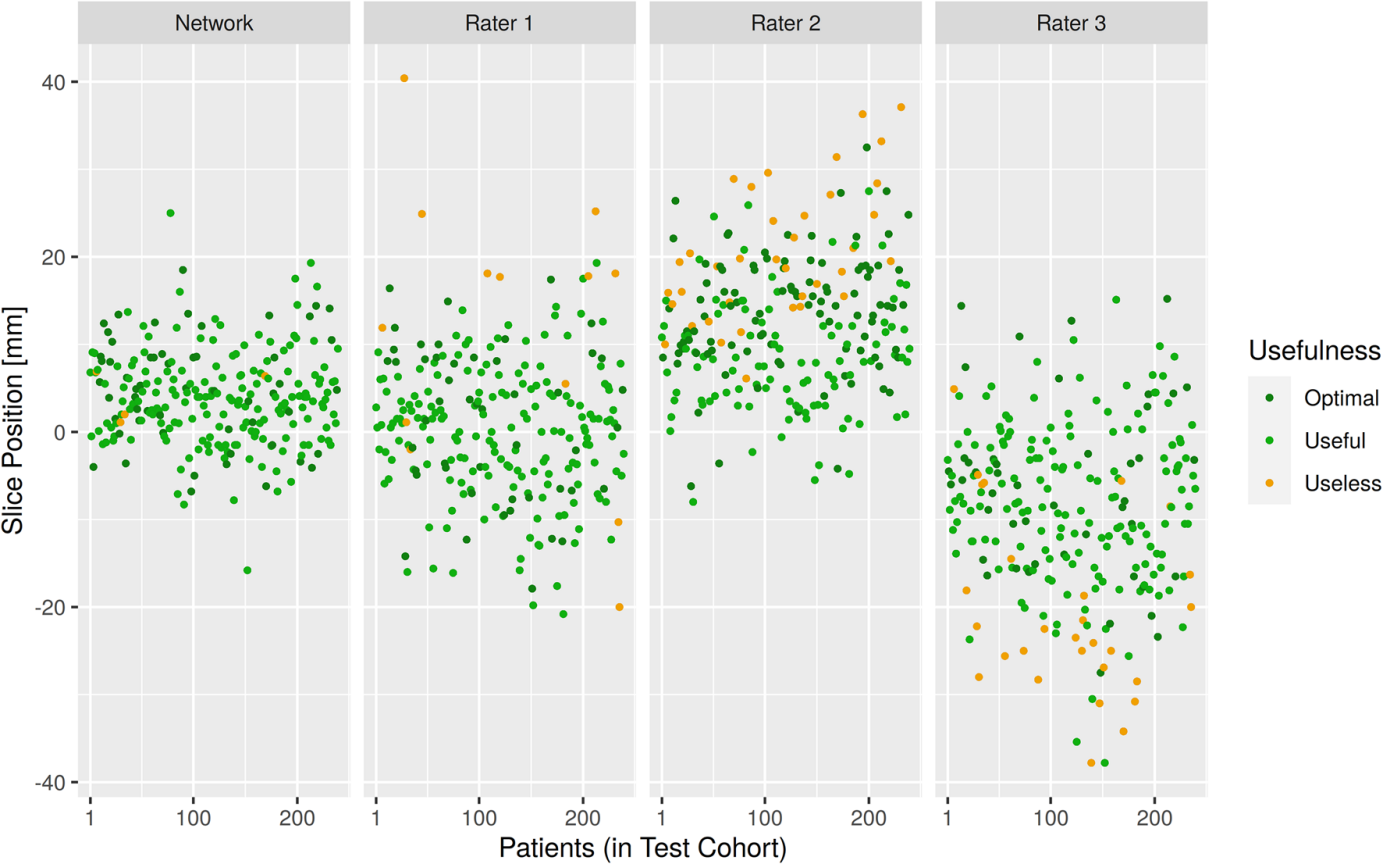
A visualization of the usefulness of the estimated slice positions of the pulmonary trunk of all radiographers and the predicted slice positions of the network on the test cohort. Slice positions are relative to the optimal slice position, which is different for each patient.
